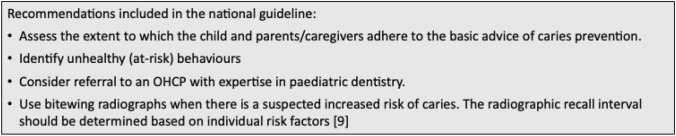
Adherence to brushing and eating advice was assessed at every visit, only during intake or only when caries was diagnosed. Caries risk factors were identified through history taking, sometimes using the ‘Gewoon Gaaf’ method. Radiograph use and frequency depended on factors like eruption of permanent teeth, caries history, caries activity, caries risk, interdental spaces and family caries. Some took a few radiographs, whilst others did so routinely at intake, at specific ages (3–7 years), or with first permanent molar eruption. Intervals ranged from 6 to 18 months depending on caries activity, or 2 to 3 years by default without caries activity. “*If everything looks good otherwise, the recall interval can be extended to two years.*” (Interview #17, dental hygienist, age 41).
